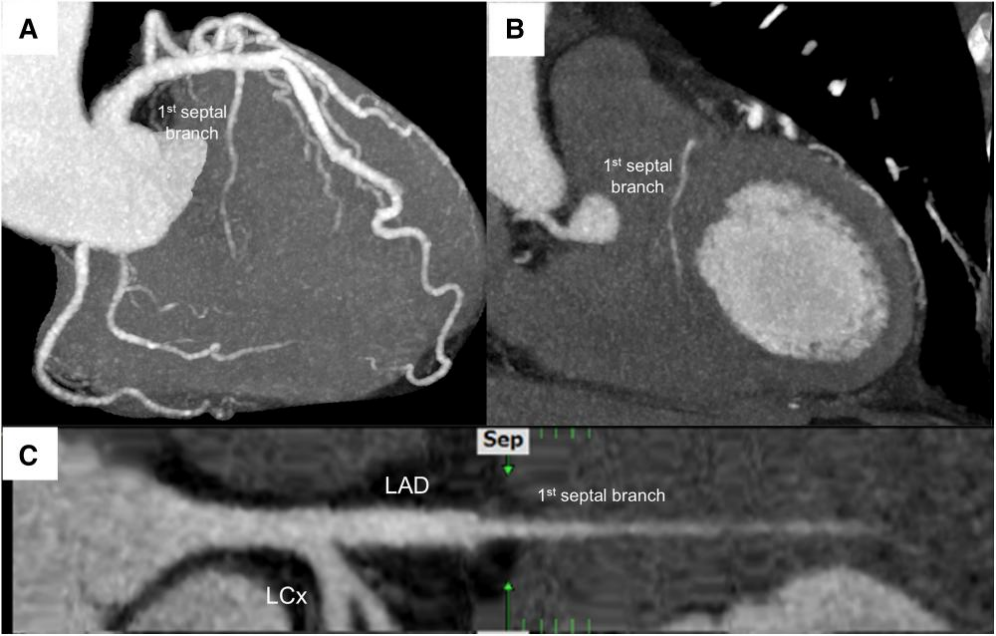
One-month follow-up coronary computed tomography angiogram. (*A*) The first septal branch shows no residual signs of dissection. (*B*) Perfused first septal branch in the infarction site. (*C*) Stretched curved planar reconstruction of the first septal branch.
